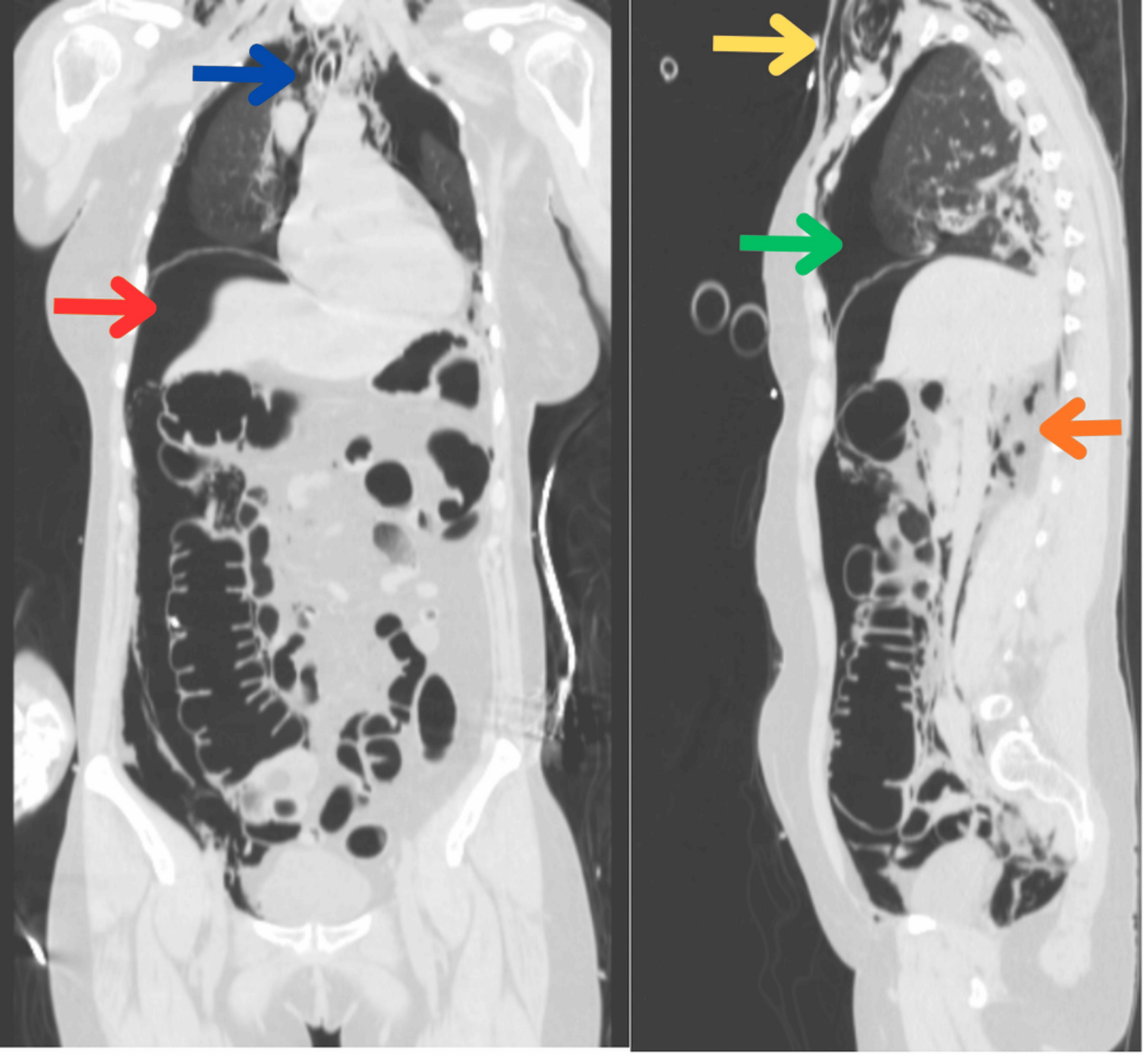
CT images: sagittal section (right) and coronal section (left). Blue arrow: pneumomediastinum; green arrow: pneumothorax; yellow arrow: subcutaneous emphysema; orange arrow: pneumoretroperitoneum; red arrow: pneumoperitoneum
